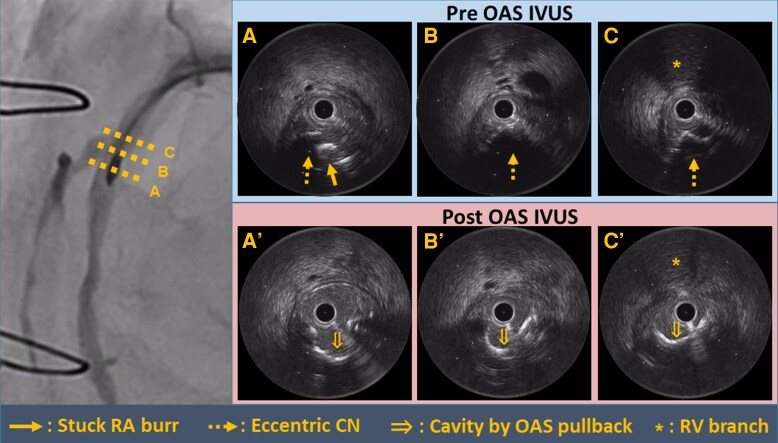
Pre- and post-orbital atherectomy system (OAS) intravascular ultrasound (IVUS) evaluation. (*A*)–(*C*) Pre-OAS IVUS demonstrates that the rotational atherectomy (RA) burr is lodged within an eccentric calcified nodule (CN) on the inner curvature of the vessel (the opposite side of the right ventricular [RV] branch). The yellow solid arrow indicates the RA burr. Yellow dashed arrows indicate the CN. Yellow asterisks indicate the RV branch. (*A*′)–(*C*′) After OAS, IVUS confirmed adequate modification of the CN, with cavity formation and significant lumen enlargement. Yellow double arrows indicate the enlarged lumen cavity.
